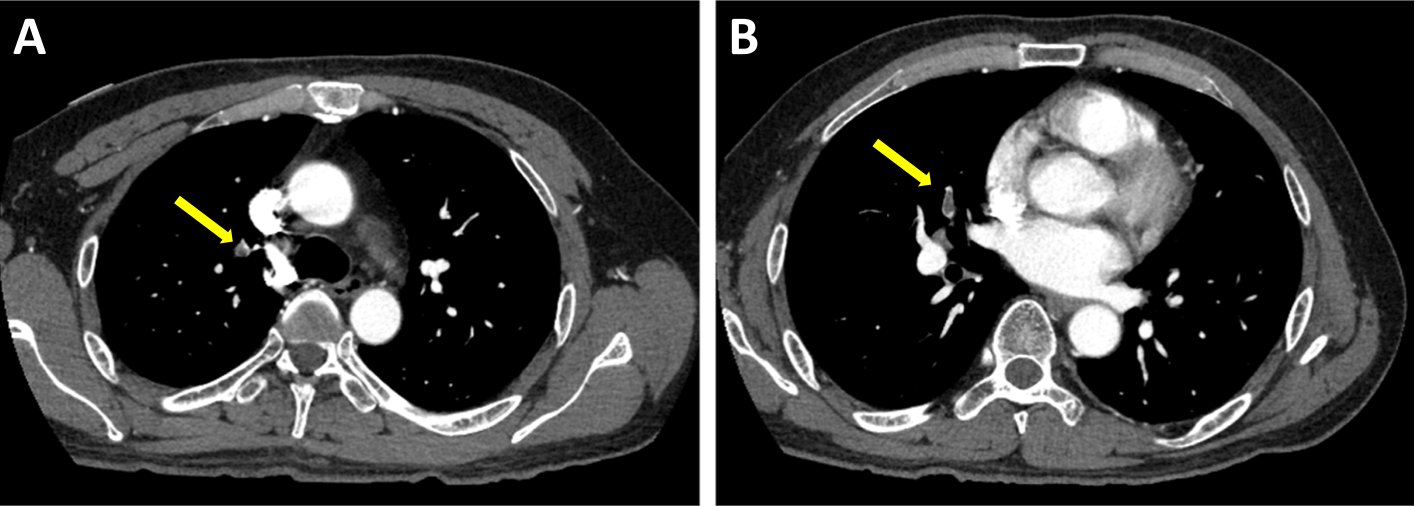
Contrast computed tomography showing small pulmonary embolism. Contrast computed tomography revealed small pulmonary embolism in the right segmental pulmonary artery (yellow arrow in a and b)
